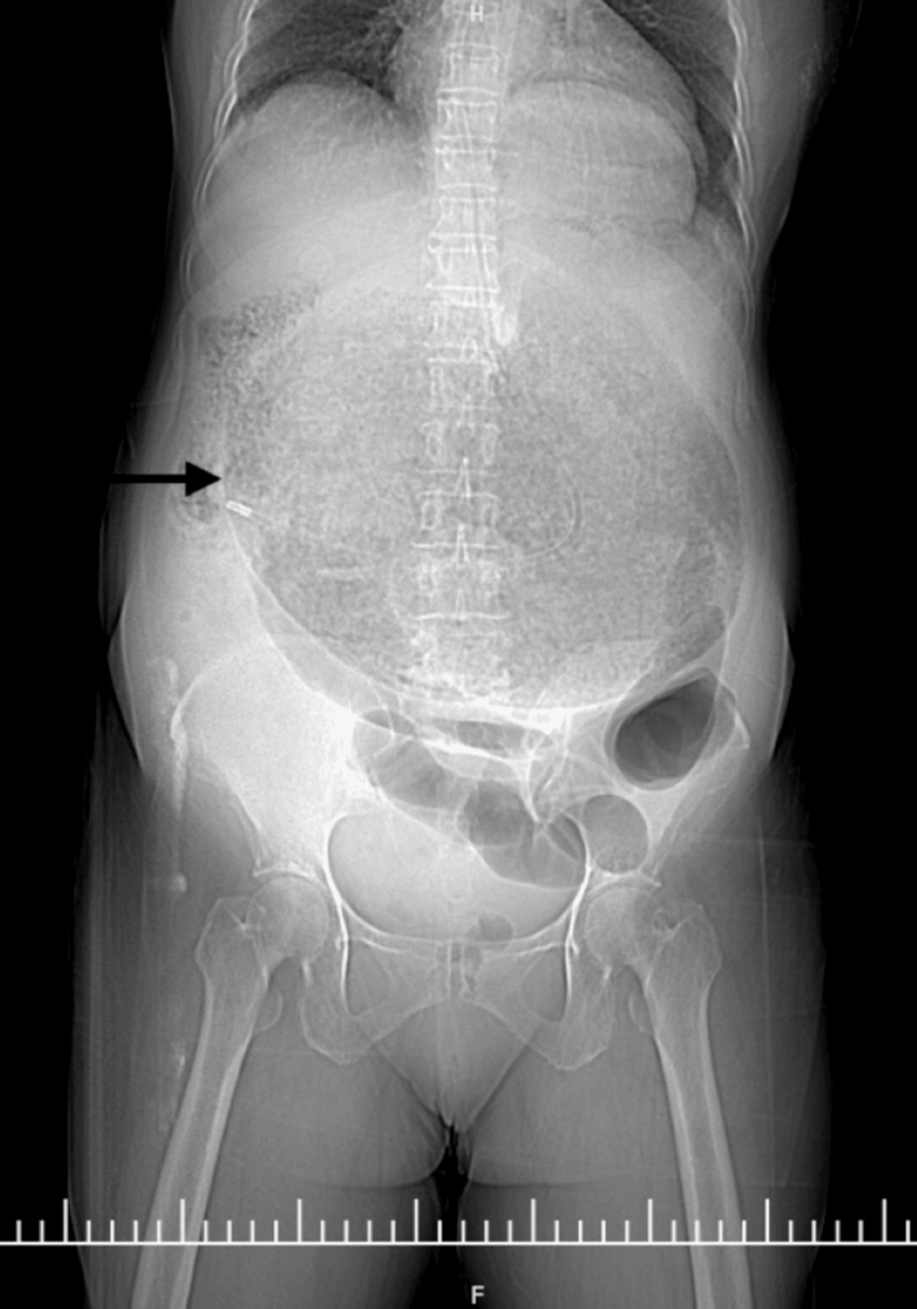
Computed tomography (CT) scout image showing a grossly distended stomach with a nasogastric tube in situ (arrow).
